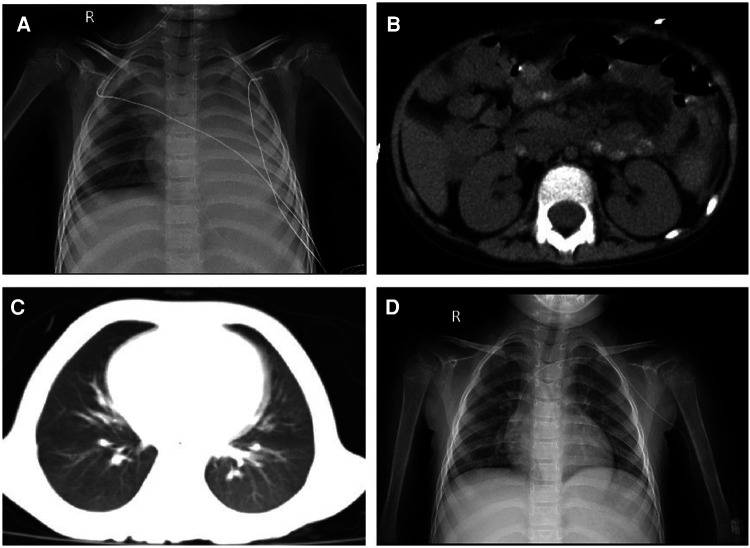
(A) Chest X-ray showing a significantly decreased transmittance in the left lung; (B) peritoneal thickening of the left paracolic sulcus; (C) chest CT showing a new exudative lesion in the post-basal segment of the left inferior pulmonary lobe; and (D) chest X-ray showing that the transmittance of the lung was improved.
